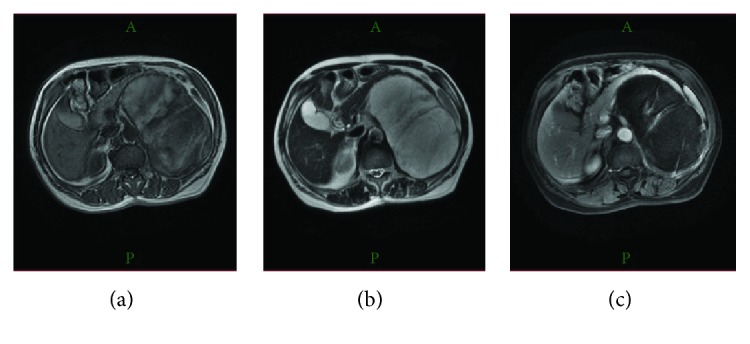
Magnetic resonance imaging (MRI) revealed a heterogeneously hyperintense mass on T1-weighted imaging (a), a relatively uniform and hyperintense mass on T2-weighted imaging (b), and a hypointense mass with an enhanced border on Gd-enhanced imaging (c).
